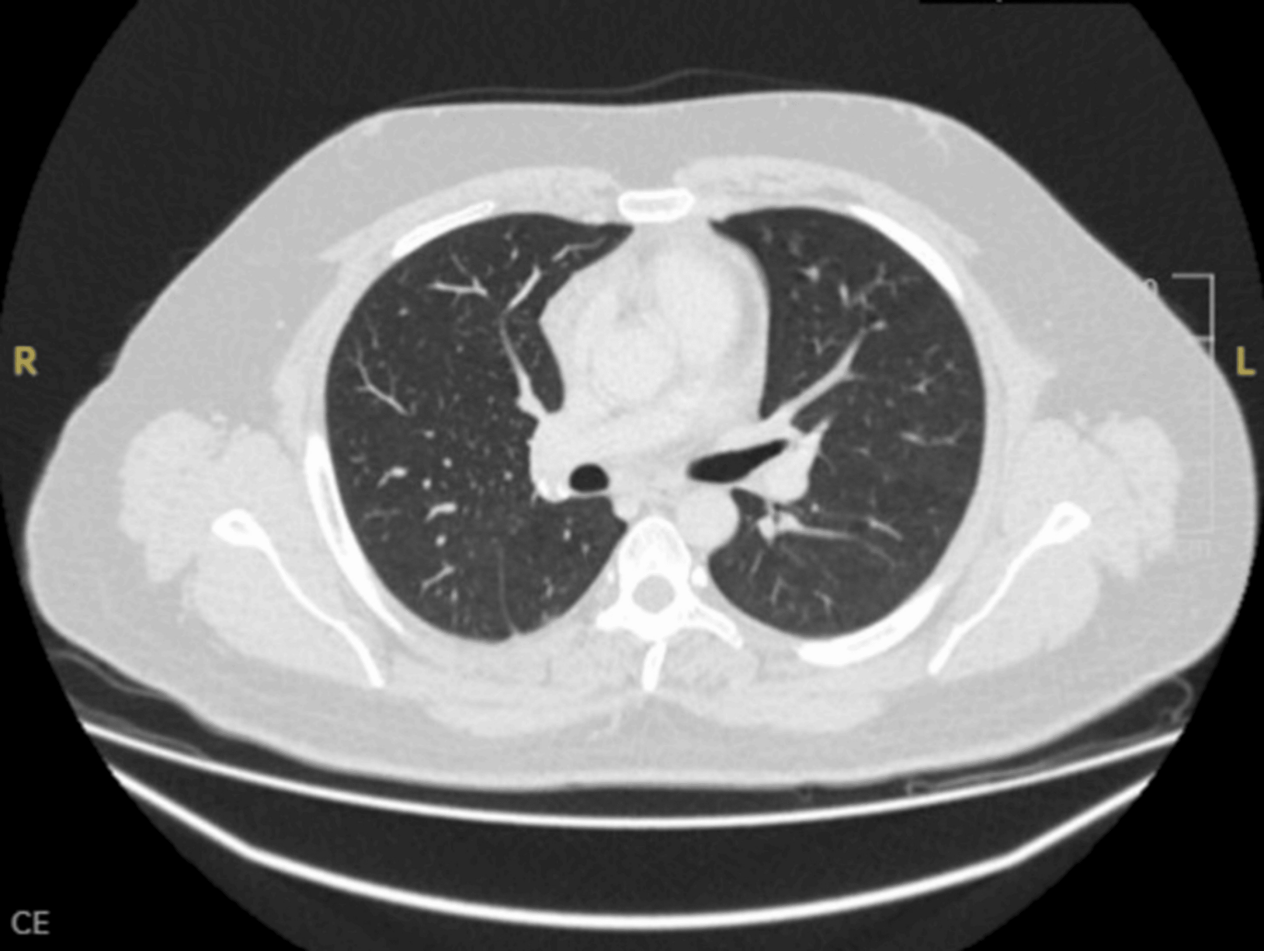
Axial CT thorax showing calcified lymph nodes in the axillary, mediastinal, and upper abdominal regions. The findings are consistent with prior granulomatous infection, such as tuberculosis. There is a noted reduction in the size of the left para-aortic lymph nodes compared to previous imaging. Lung parenchymal changes appear stable. CT: computed tomography
